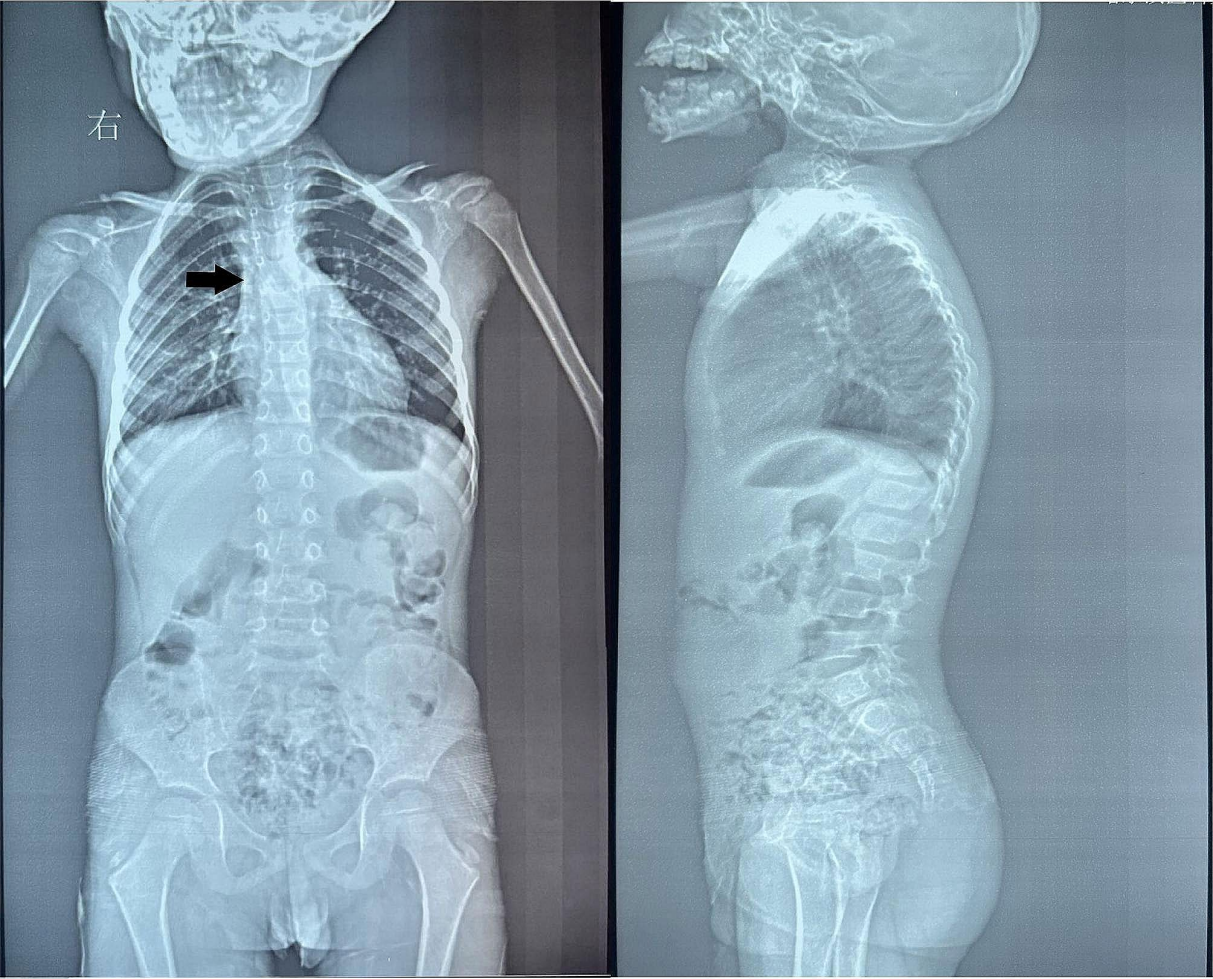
The spine radiograph (black arrow) showing scoliotic spine
